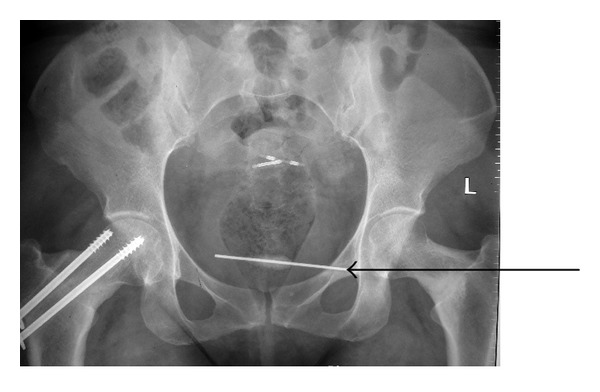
Photograph of the X-ray of pelvis showing the K-wire in the pelvis. A previously inserted copper-T in the uterus is also seen.
